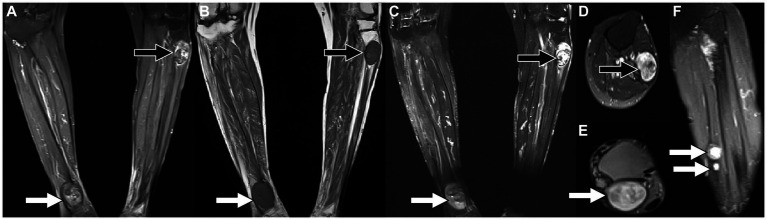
Magnetic resonance imaging [MRI; (A) T_2_-weighted imaging (T_2_WI); (B) T_1_-weighted imaging (T_2_WI)] reveals that there are fusiform soft tissue masses with low signal on T_1_WI and high signal on T_2_WI under the skin of the proximal tibia on the left and the distal tibia on the right, of which boundaries are clear. Contrast-enhanced T1WI sequence scan after contrast agent injection [(C) Coronal; (D,E) Axial; (F) Sagittal view shows small nodules of markedly enhanced soft tissue in the middle of the left calf (arrows)] revealed significant enhancement in both lower extremity lesions.
